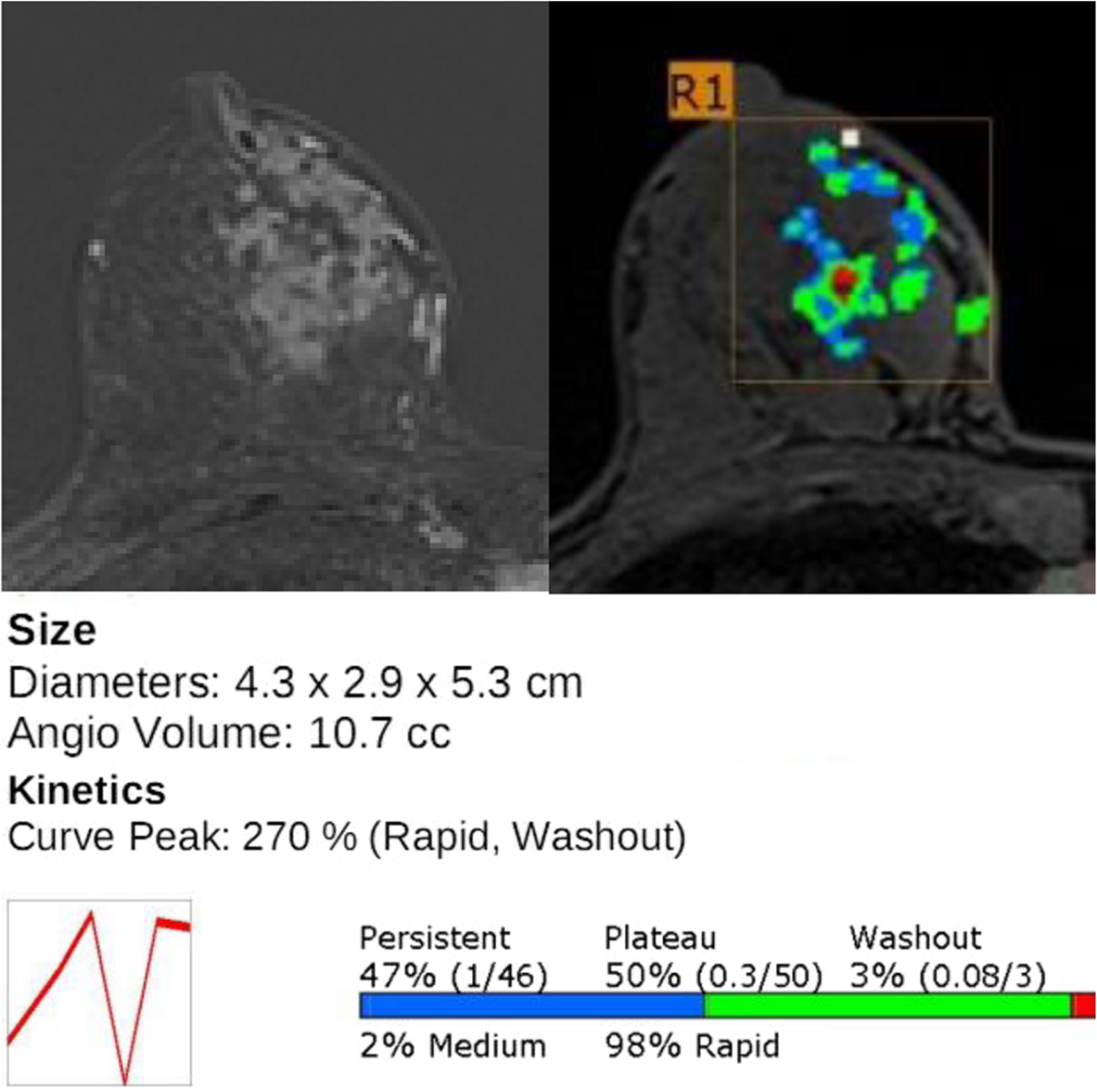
Imaging features of invasive ductal carcinoma on final surgical pathology. Axial fat-suppressed T1-weighted contrast-enhanced MRI showed a segmental distributed non-mass enhancement with clustered ring enhancement (left). MRI with a CAD color overlay map indicated the tumor size and enhancement kinetics, i.e., a 270% peak enhancement and a 3% washout component (right)
